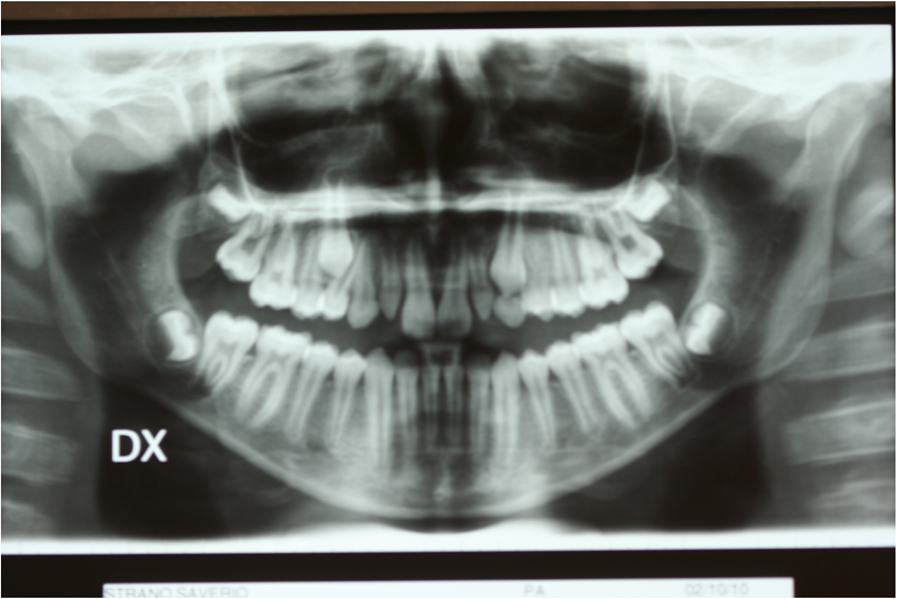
Pretreatment orthopantomography.
